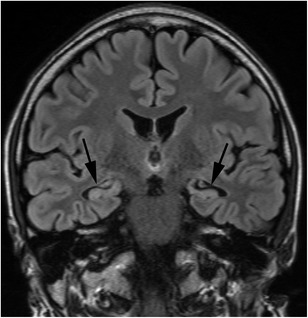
Hippocampal sclerosis. A 24-year-old-man, who received bone marrow transplantation for malignant lymphoma at the age of six, presented with temporal lobe epilepsy. FLAIR image shows atrophy with high signal intensity in the bilateral amygdalae and hippocampi (*arrows*)
